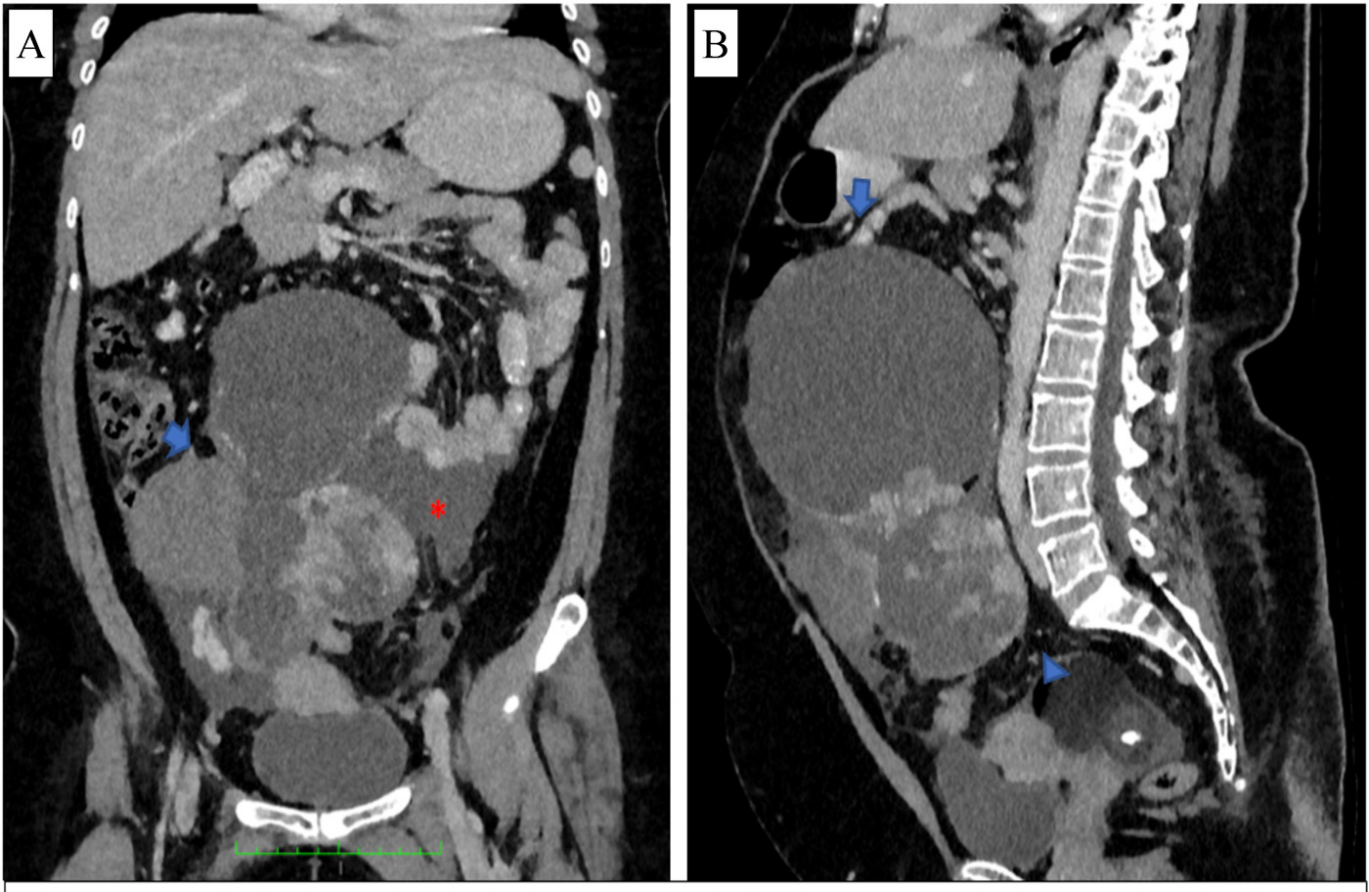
Abdominal multi-slice CT (MSCT): (A) coronal and (B) sagittal views in the venous phase, demonstrating adnexal masses on the right ovary (arrow) and left ovary, which have cystic, fat, solid, and calcification components. The solid component shows contrast enhancement, and ascites in the abdominal and pelvic cavities (asterisk).
